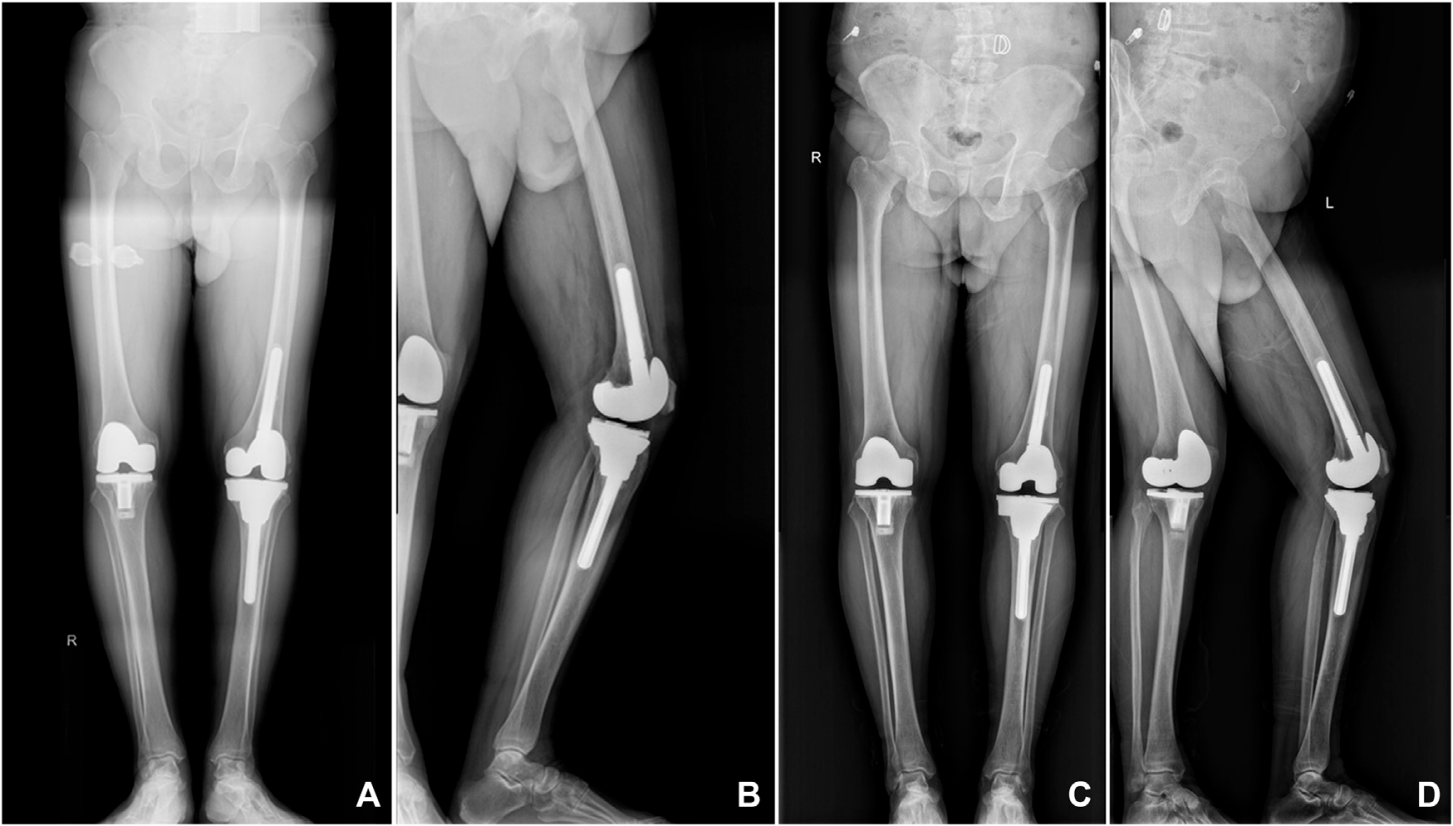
X-ray images of a representative patient showed that the 3D-printed porous tantalum cone is stable and tightly integrated with the surrounding bone tissue. (A,B) Postoperative radiographs were taken at 6 months after surgery; (C,D) postoperative radiographs were taken at 3 years after surgery.
